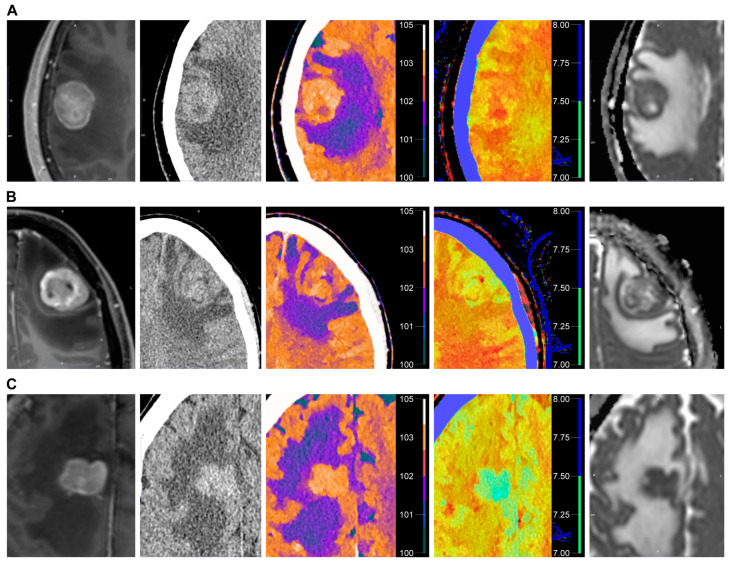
Representative images from a 57-year-old woman with brain metastasis of breast cancer (A), a 38-year-old woman with glioblastoma, IDH-wildtype (B), and a 74-year-old man with diffuse large B-cell lymphoma (C). From left to right, contrast-enhanced T1-weighted image, conventional 120-kVp CT, electron density map, effective atomic number map, and apparent diffusion coefficient map.
